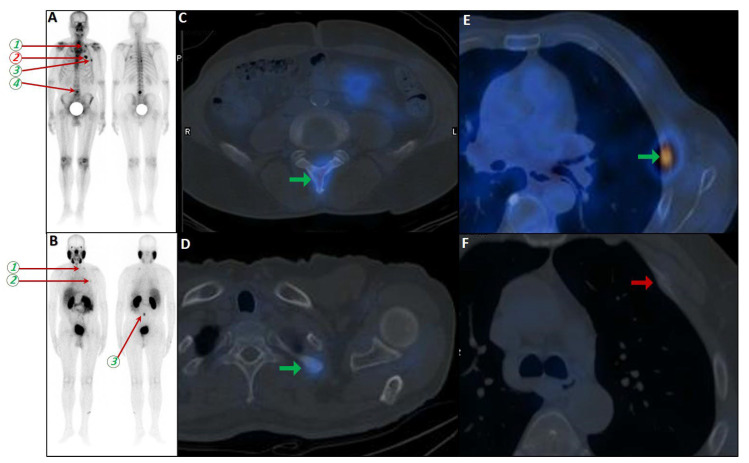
Partial concordance of PSMA and bone scan. A 66-year-old man with prostate adenocarcinoma (biopsy Gleason score 4 + 5 in 8/12 cores, serum PSA = 45 ng/dL) was referred for staging. Bone scan showed four foci of increased osteoblastic activity, three in the ribs (non-consecutive, anterior/lateral aspect) on the left side as well as L5 vertebra (A). Whole-body 99mTc-PSMA scan SPECT/CT (B) showed PSMA-avid lymph node metastases in the left external iliac and left common iliac (not shown). Skeletal metastases (green arrow) in the L5 spinous process (C) and posterior aspect of the left 2nd rib (D) and lateral 5th left rib (E), and non-PSMA-avid fracture in the 4th left rib (red arrow, (F)). The patient was down-staged from polymetastatic high-volume disease to oligometastatic disease.
